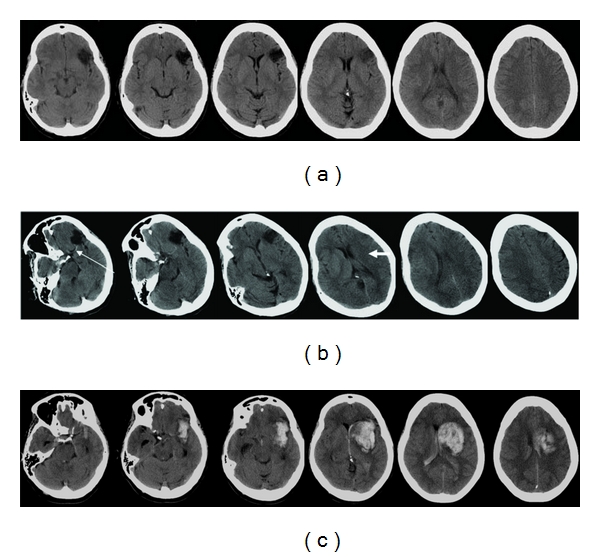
74-year-old woman presented with right hemiparesis lasting few minutes but ongoing residual sensory deficits. (a) Initial axial unenhanced CT images show an old left frontal infarct only. (b) 12 hours after her initial symptoms, she developed recurrent right hemiparesis and aphasia. Repeated axial-unenhanced CT images show obscuration and loss of grey white differentiation in the left lentiform nucleus (thick arrow) and a dense left middle cerebral artery (thin arrow). (c) Axial unenhanced CT images 24 hours after stroke show a large left parenchymal hematoma within the striatocapsular region. Importantly, even in the absence of the previous CT studies, the involvement of the caudate head in the last series of CT images raises the possibility of hemorrhagic infarct. Stage IIH d2 [1].
